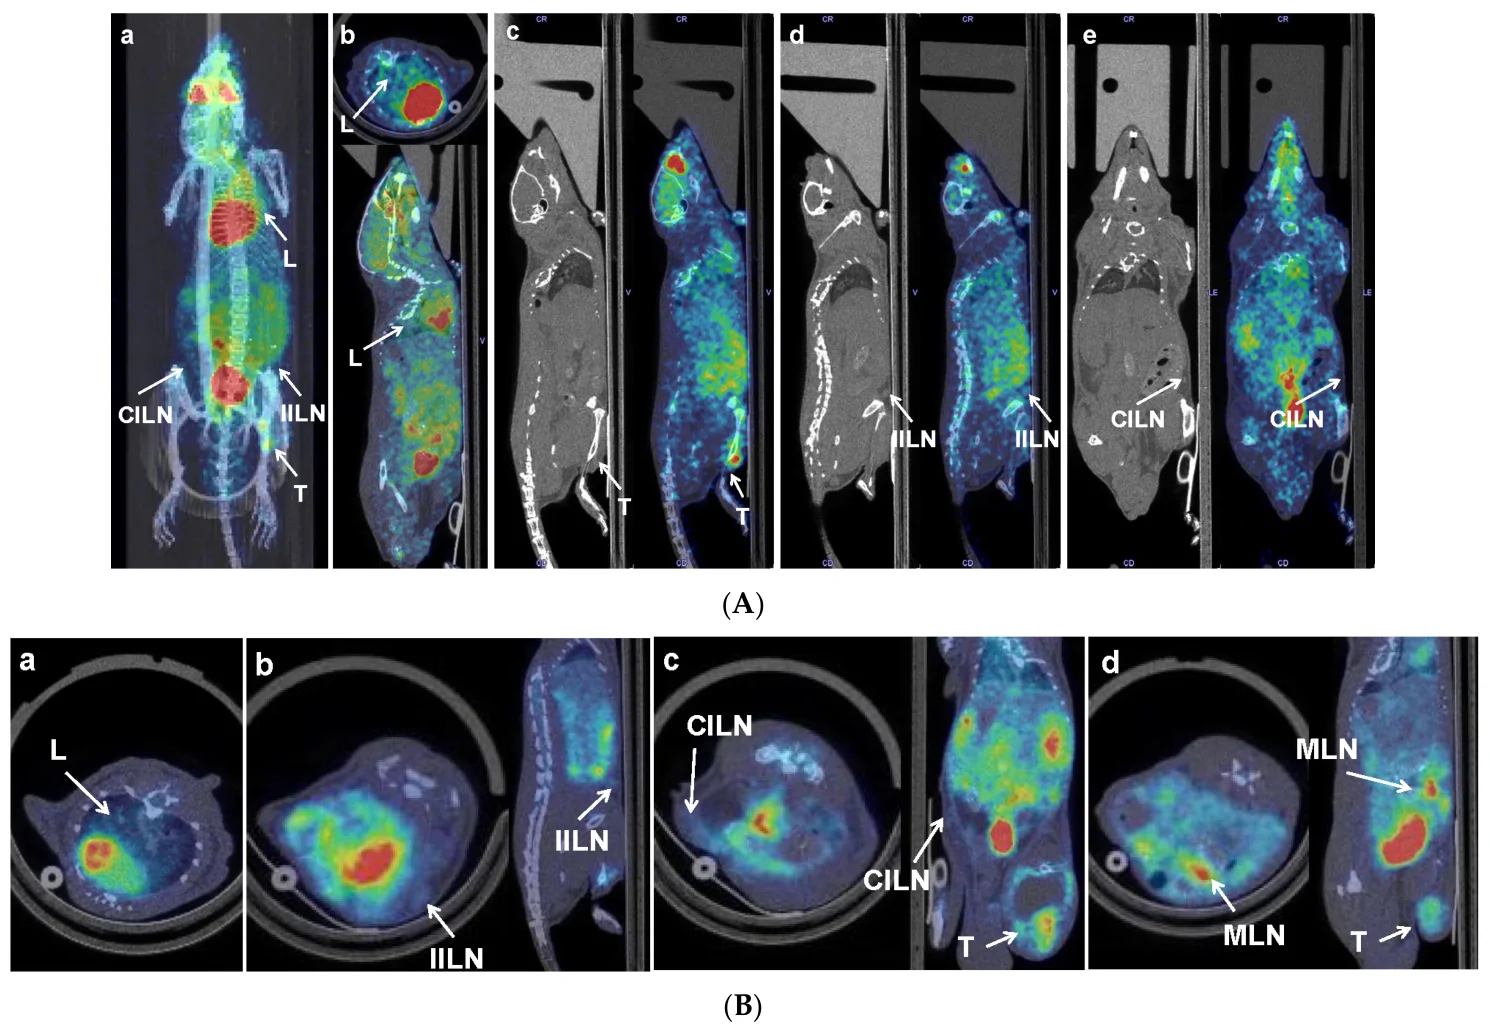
(A) ^18^F-FDG PET/CT imaging revealed upregulated signals in tumor (T), lungs (L), and ipsilateral inguinal lymph node (IILN), but not the contralateral inguinal lymph node (CILN) at 10 days post tumor cell implantation. (a): whole body; (b): transverse and sagittal; (c): Sagittal CT and PET/CT; (d): Sagittal CT and PET/CT; (e): Coronal CT and PET/CT. (B) 18F-FDG PET/CT imaging revealed upregulated signals in tumors (T), lungs (L), ipsilateral inguinal lymph node (IILN), contralateral inguinal lymph node (CILN), and mesenteric lymph node (MLN) at 18 days post tumor cell implantation. (a): transverse; (b): transverse and sagittal; (c): transverse and coronal; (d): transverse and sagittal.
